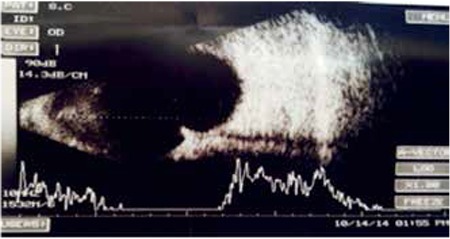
Ultrasonography of the inferotemporal quadrant showing hyperechogenic appearance of the Arruga suture invading the vitreous
